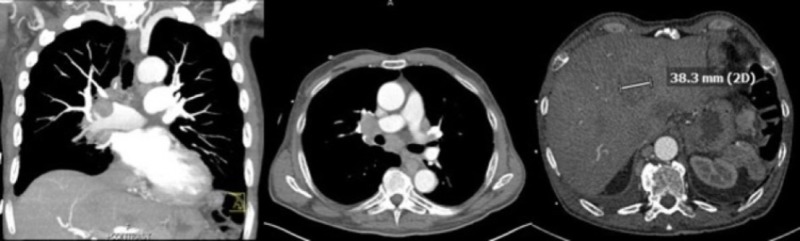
Computed tomography angiography (CTA) of the chest CTA imaging of the chest revealing a conglomerate right hilar mass involving the carina and extending inferiorly into the subcarinal, hilar, and paratracheal regions with encasement of the right main stem bronchus, bronchus intermedius, and right pulmonary artery. Also pictured is evidence of superimposed pneumonia, as well as retroesophageal and mediastinal lymphadenopathy with findings suspicious for hepatic and retrocaval metastasis.
